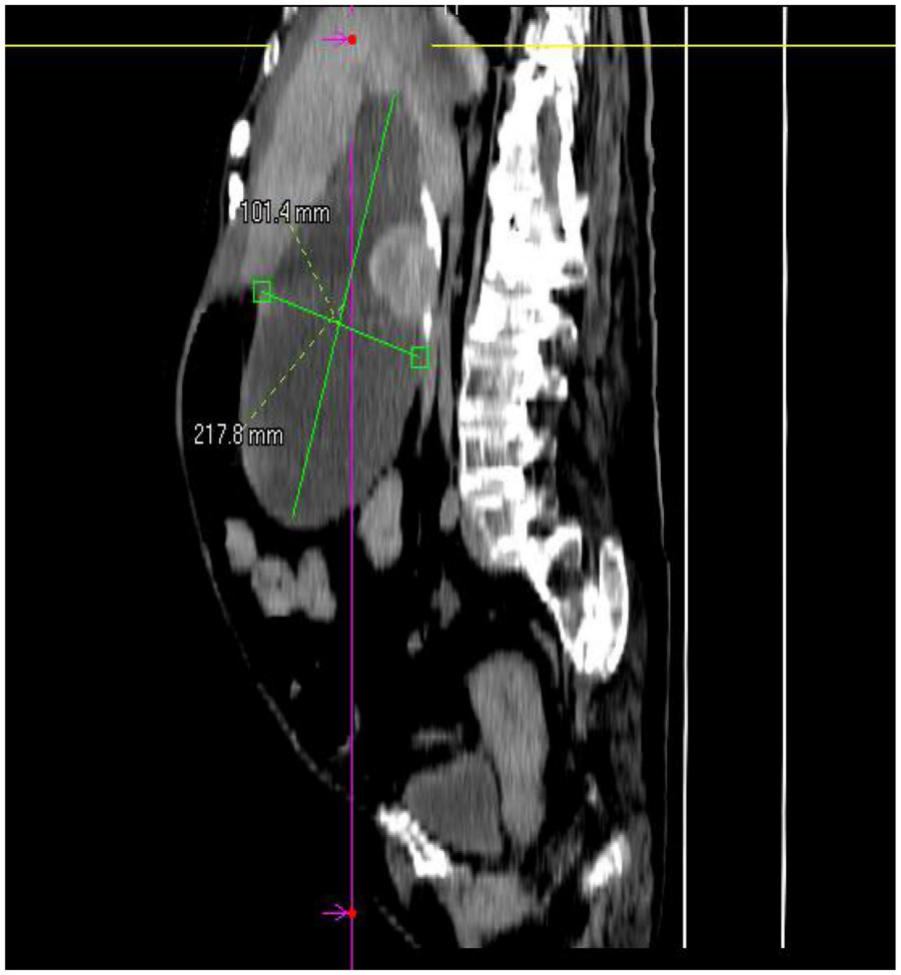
Whole-abdominal CT shows: the gallbladder has a long diameter of 217.8 mm and a transverse diameter of 101.4 mm.
